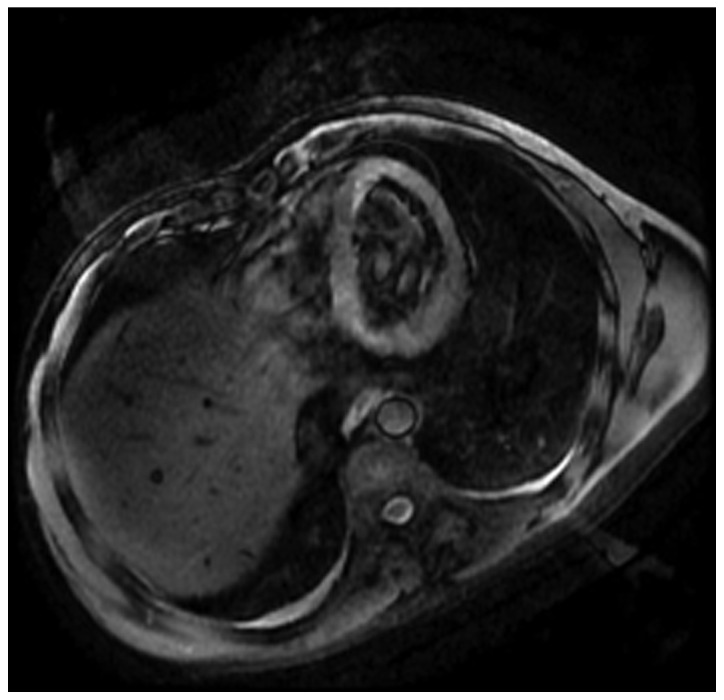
Patient observed using cardiovascular magnetic resonance imaging, the circle indicates the left ventricular anterior wall where a long T2 signal was observed.
